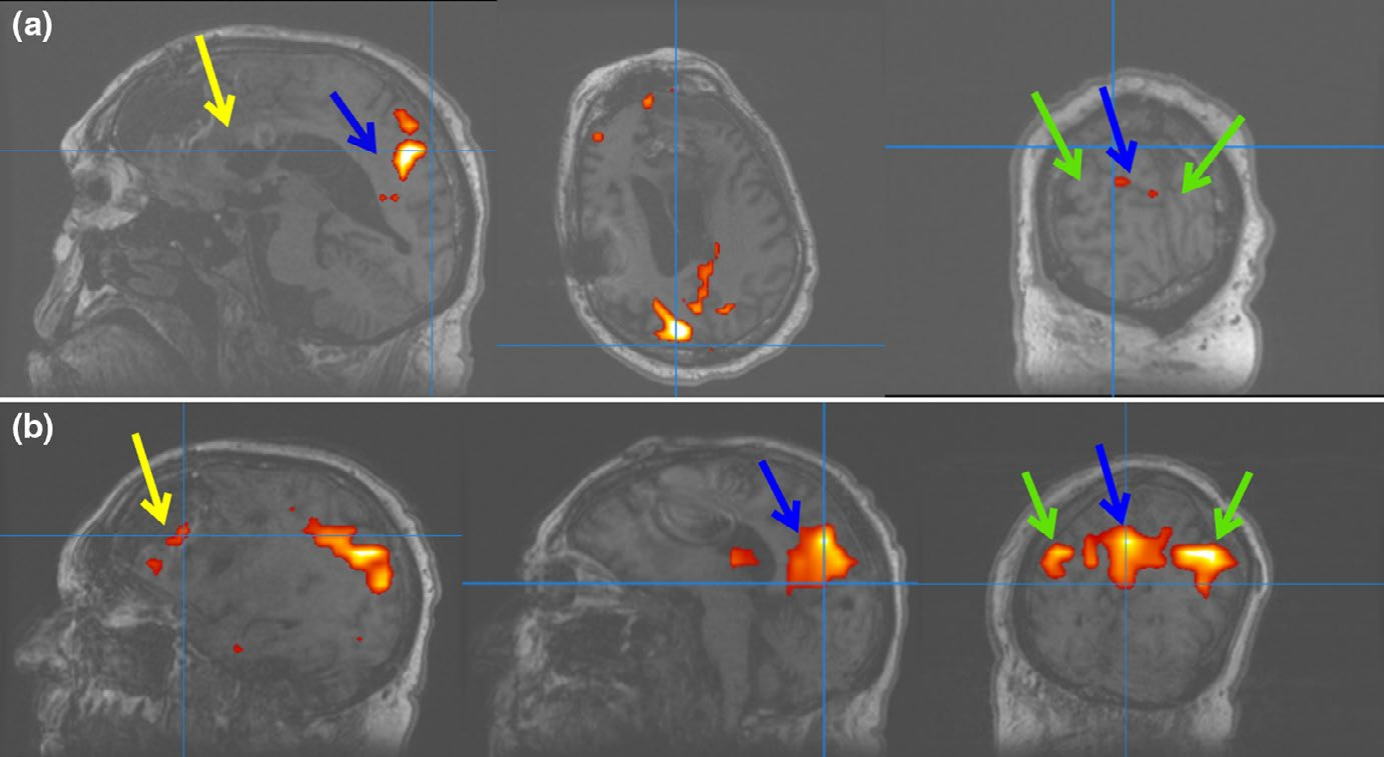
Resting‐state functional magnetic resonance imaging data demonstrating the independent component analysis (ICA) of the default mode network (DMN) in the patient (a) pretranscranial magnetic stimulation (TMS) and (b) post‐TMS. Blue arrows represent the posterior cingulate cortex, green arrows represent inferior parietal cortex, and yellow arrows represent the anterior cingulate cortex, wherein the DMN components would be expected to be seen. There was no clear evidence of the DMN in the pre‐TMS (a) ICA analysis as demonstrated by no components near the arrows. The closest component in the pre‐TMS (a) analysis near the posterior cingulate cortex seems to represent the default attention network, not the DMN. Post‐TMS (b), components representing the DMN appeared, as seen by the red areas of activity demonstrated by the arrows
